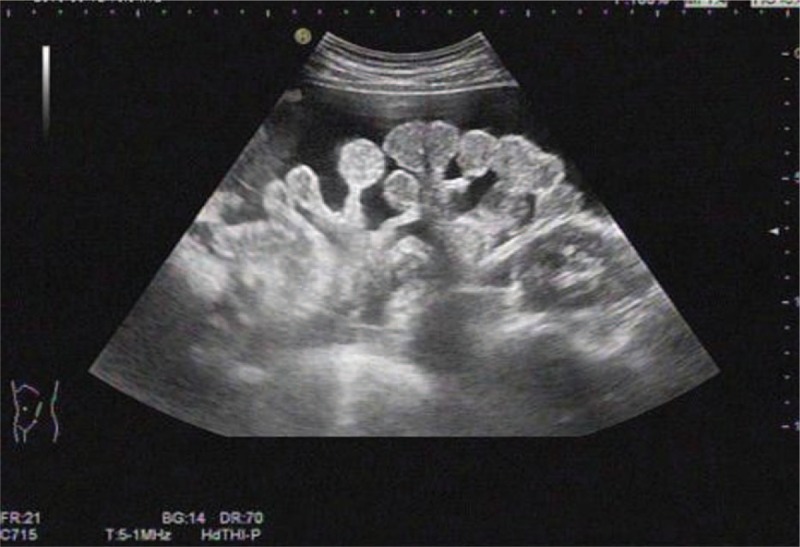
An abdominal ultrasound showing massive ascites.
